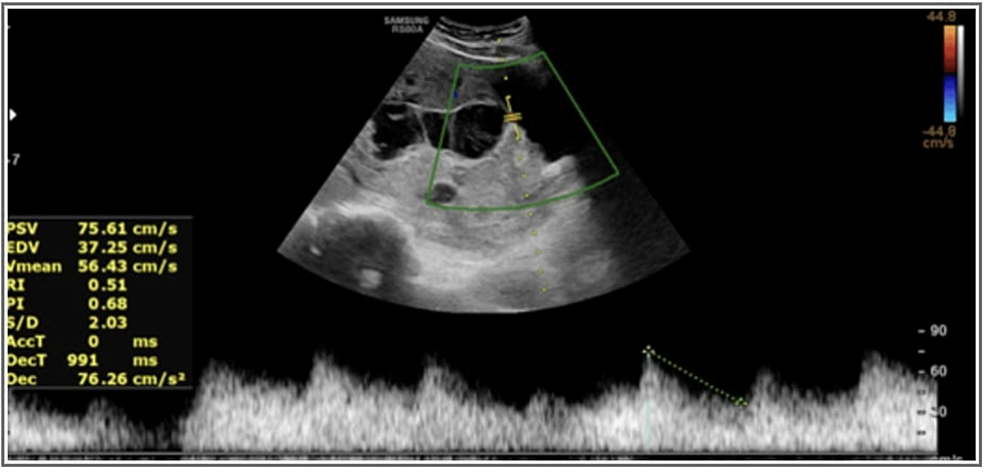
USG Doppler scan image USG: ultrasonography
